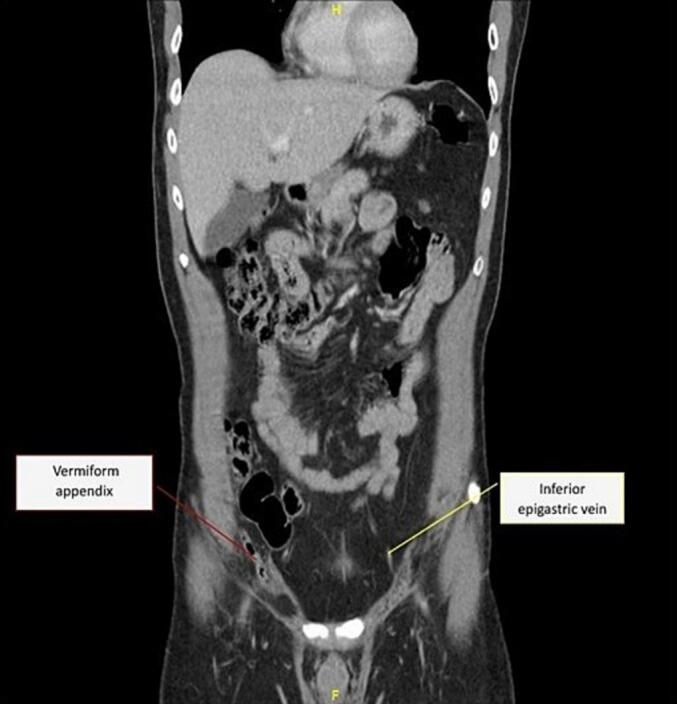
CT abdomen coronal view showing herniated appendix (red label). (For interpretation of the references to color in this figure legend, the reader is referred to the web version of this article.)
